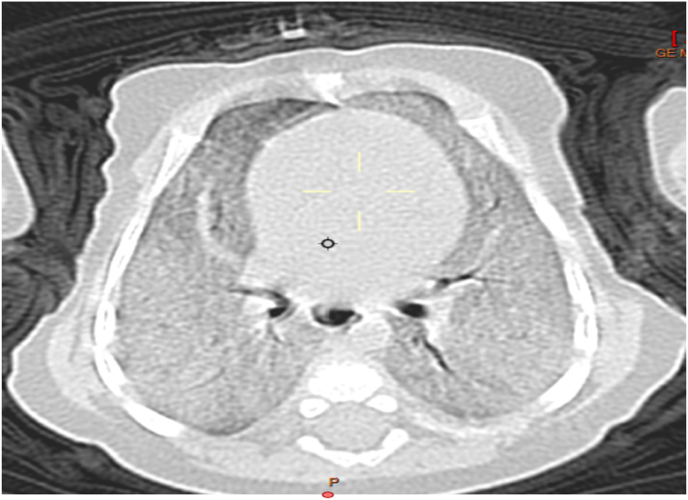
Thoracic CT scan showing lung damage of 95%.
